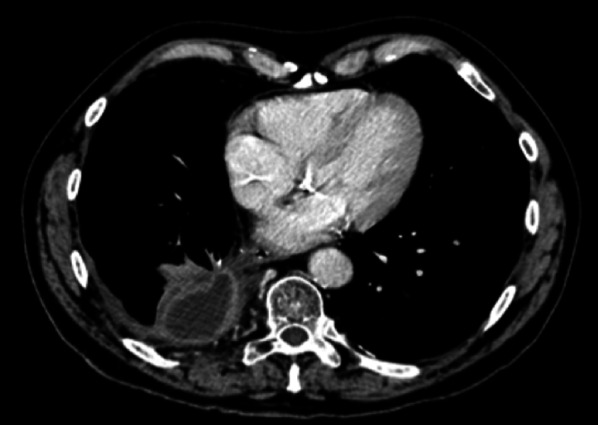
Pleural empyema. Axial post-contrast CT image (mediastinal window) showing a pyothorax caused by a bronchopleural fistula in the aftermath of a right upper lobectomy. We can observe loss of the internal concavity of the pleural pouch, with a biconvex collection, a thickening of the pleural pouch wall with parietal enhancementC.
